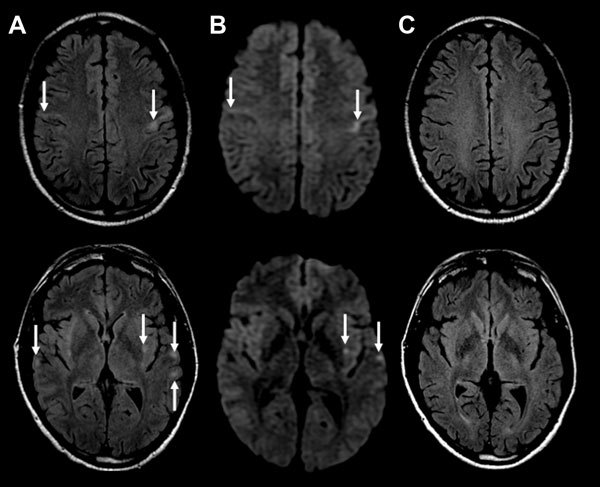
MRI findings from a 47-year-old man with encephalitis-associated human metapneumovirus pneumonia, Australia. A) Axial MRI FLAIR at presentation. Arrows indicate multiple areas of bilateral subcortical and external capsule FLAIR hyperintensities and perirolandic predominance (top image). B) Axial MRI DWI at presentation. Arrows indicate corresponding increase in DWI signal in the affected areas. C) Axial FLAIR MRI after 3 months. The MRI changes have all resolved. DWI, diffusion weighted imaging; FLAIR, fluid-attenuated inversion recovery; MRI, magnetic resonance imaging.
